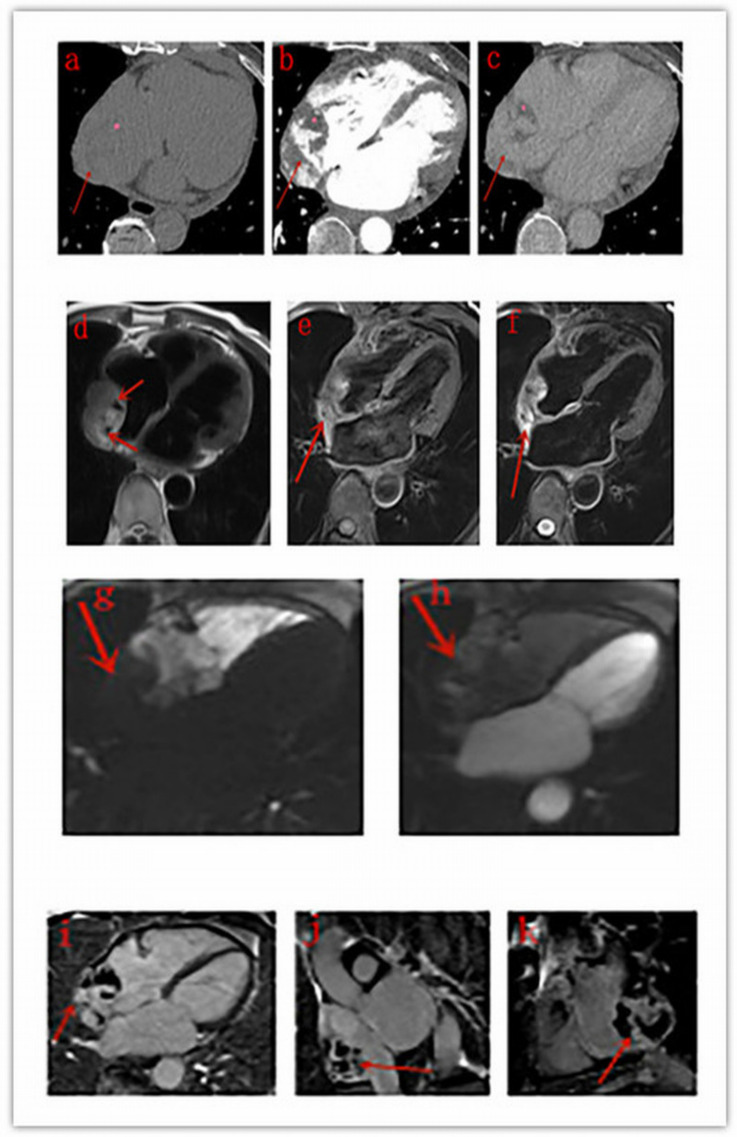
Male, 74 years old, right atrium angiosarcoma. (a–c): The mass (red arrow) in the right atrium presents as inhomogeneous density with low-density areas on plain CT scans (a) and shows strong heterogeneous centripetal enhancement on enhanced CT scans (b,c), and the low density area (pink star) shows no enhancement. (d–f): Signal void (red arrow) is noticed on a black-blood haste sequence (d). The mass (red arrow) presents as heterogeneous hyperintensity on T1WI (e) and T2WI (f). (g–h): On first-pass perfusion sequences, the mass (red arrow) presents as a filling defect in the right atrium as the contrast medium transits the right side (g) and is enhanced as the medium goes through the left side (h). (i–k): LGE shows heterogeneous patchy enhancement (red arrow) on different planes.
